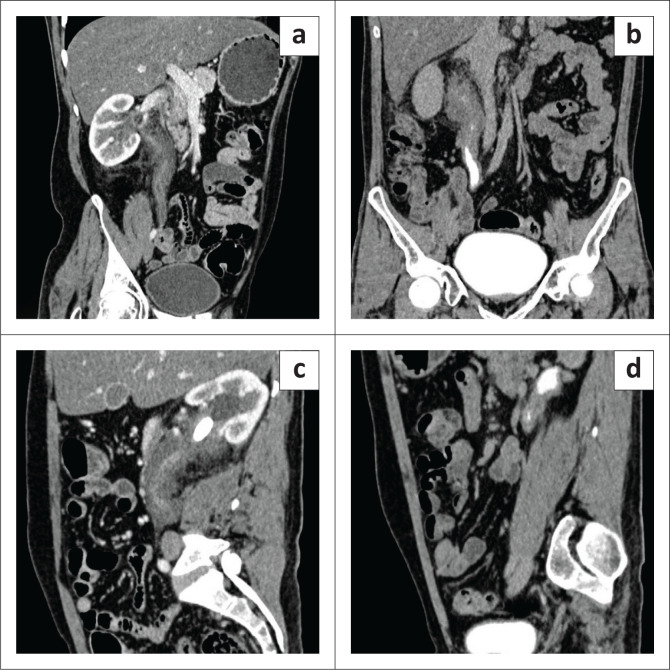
Coronal reformatted (a–b) and sagittal (c–d) sections of the contrast CT abdomen of case 1 show (a–c) a circumferential cuff of non-enhancing soft tissue mass encircling the right upper ureter and renal pelvis, extending for a length of approximately 12 cm along the upper ureter; (d) cuff of soft tissue mass involving the left upper ureter causing luminal effacement.
